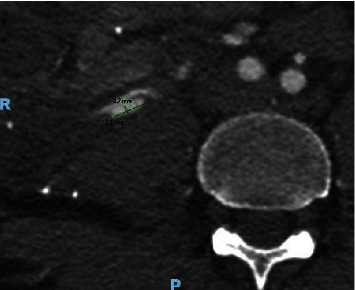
Contrast computed tomography scan of the abdomen showing one of the two pseudoaneurysms of the superior artery of the *Y*-graft.
